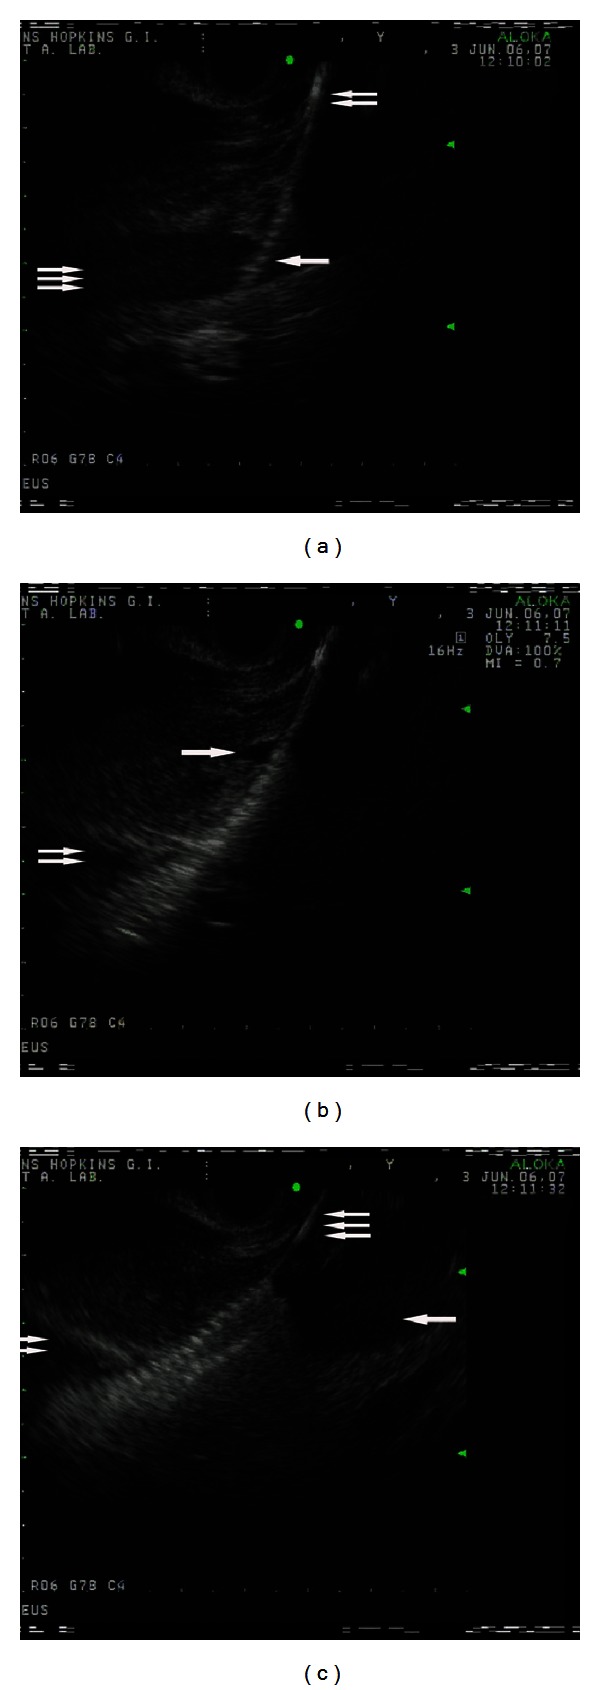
Endosonographic views during the stent deployment. (a) The stent (one arrow) delivery over the guidewire (two arrows) into the hepatic vein (three arrows). (b) Deployment of the stent; the proximal end of the stent inside the hepatic vein (one arrow) and the distal end of the stent inside the portal vein (two arrows). (c) The stent fully deployed with its proximal end inside the hepatic vein (one arrow) and the distal end inside the portal vein (two arrows); the guidewire (three arrows) (images curtsey of [51]).
